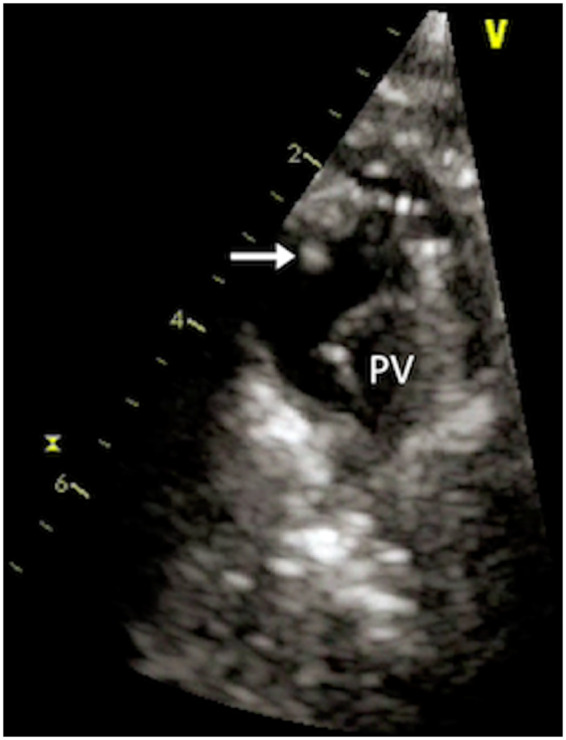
Same patient of *Figure 1* after 6 weeks of antibiotic treatment (Case 1). Transthoracic echo showed significant vegetation size reduction. Arrow, vegetation; PV, pulmonary valve.
